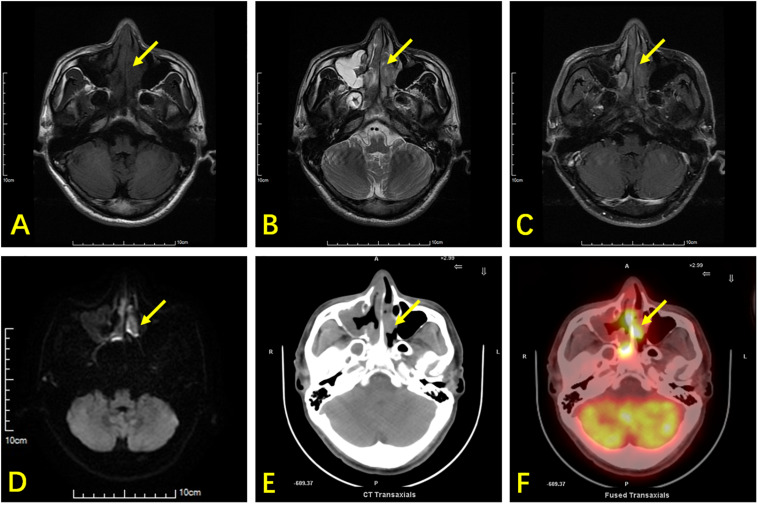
A 42-year-old man with ENKTL presented with nasal obstruction. (A) Axial section T1WI shows an isointense soft-tissue mass (arrow) in the nasal cavity and nasal septum. (B) Axial section T2WI shows the abnormal tissue as heterogeneously hyperintense. (C) Contrast-enhanced T1WI showing heterogeneous enhancement of tumor tissues. (D) DWI shows limited lesion diffusion. (E) Axial CT shows a soft tissue mass and nasal septum destruction. It was difficult to distinguish the soft tissue mass from inflammatory thickening of the mucosa in the right maxillary sinus using CT. (F)
